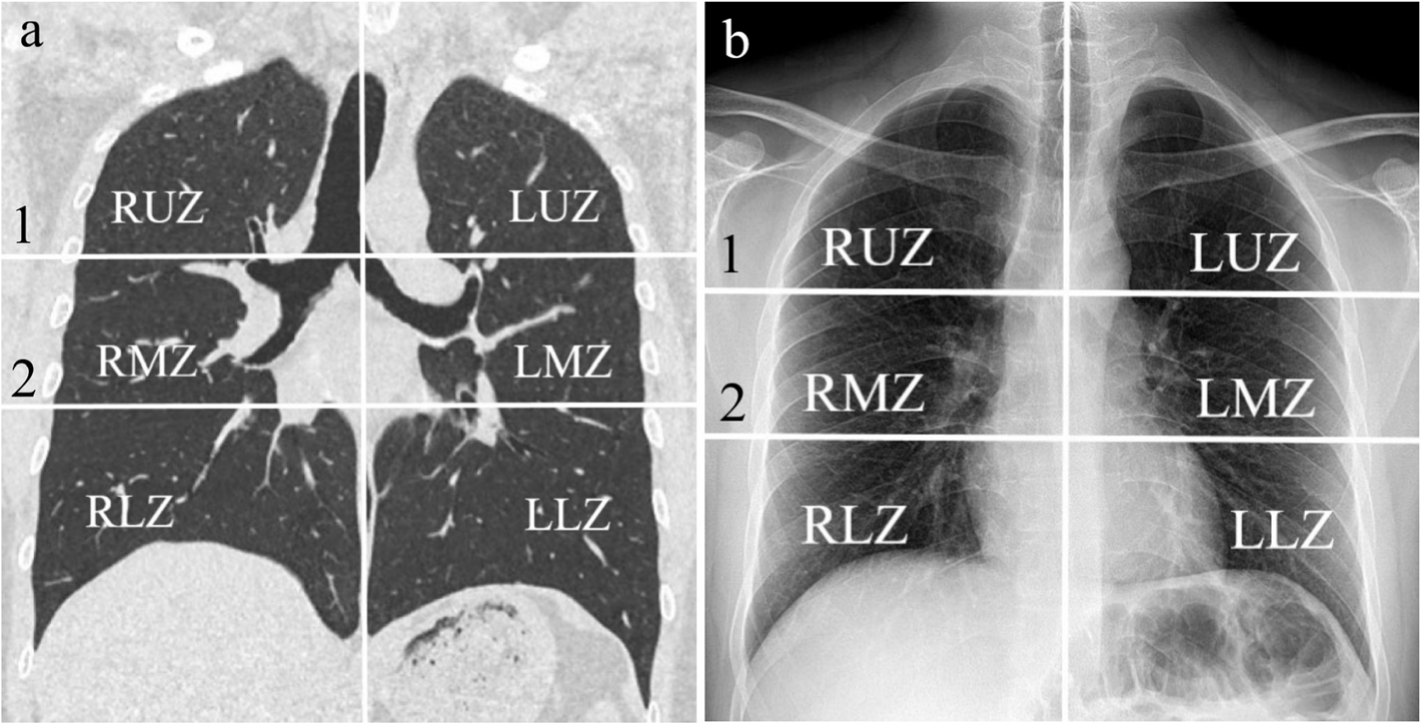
a, b Lung zones. Two lines, one drawn at the carina level (1) and the other drawn at the level of the inferior wall of the right inferior pulmonary vein (2) divide the lung into 6 zones on both coronal reformatted CT (a) and CXR (b) images. *LLZ* left lower zone, *LMZ* left middle zone, *LUZ* left upper zone, *RLZ* right lower zone, *RMZ* right middle zone, *RUZ* right upper zone
